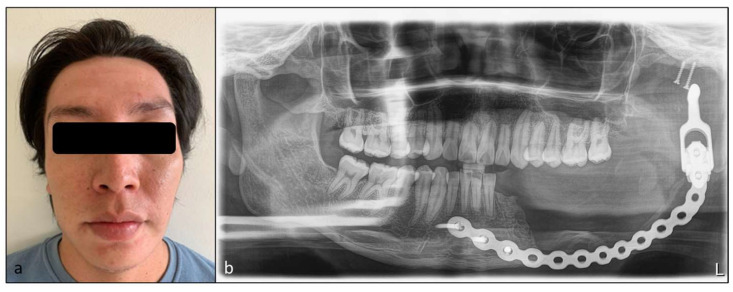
Clinical and imaging status at 15 months of following. (a) Extraoral photography of patient, showing symmetric facial appearance without the presence of tumoral remanent. (b) Panoramic radiography confirming the absence of tumoral remanent and the correct stability of reconstruction bar and personalized PMMA glenoid cavity.
